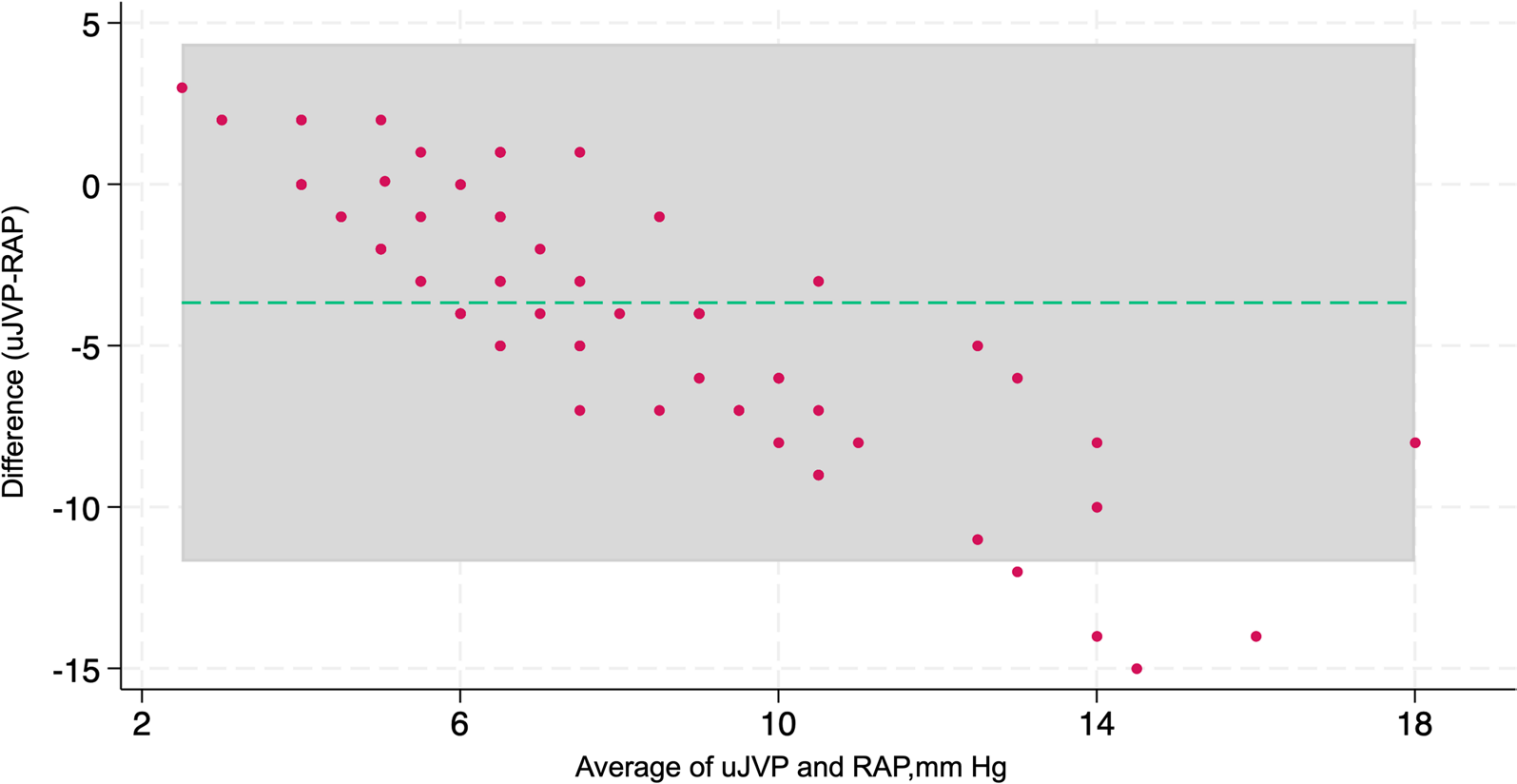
Bland–Altman Plot of uJVP and RAP; *uJVP* ultrasound-measured Jugular Venous Pressure, *RAP* Right Atrial Pressure
